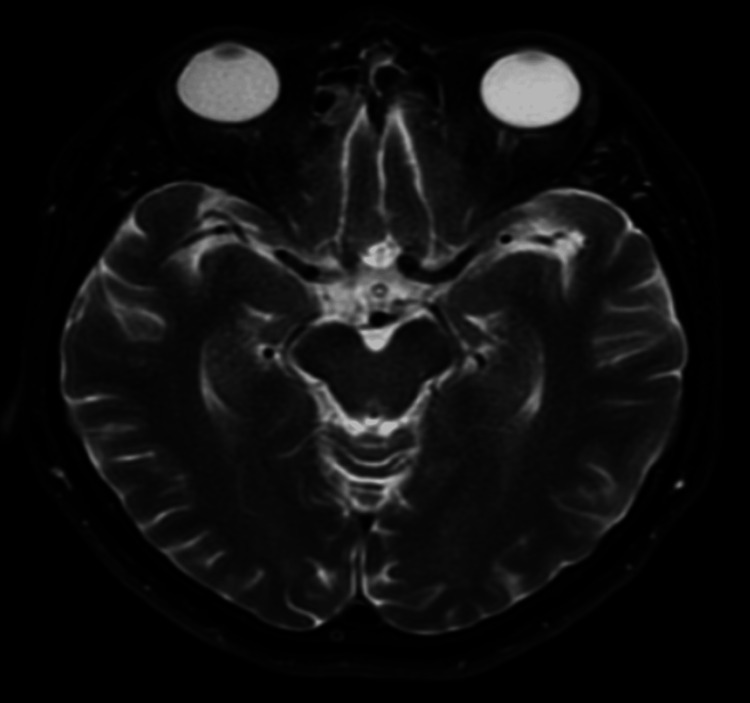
Axial view MRI T2-weighted sequence
